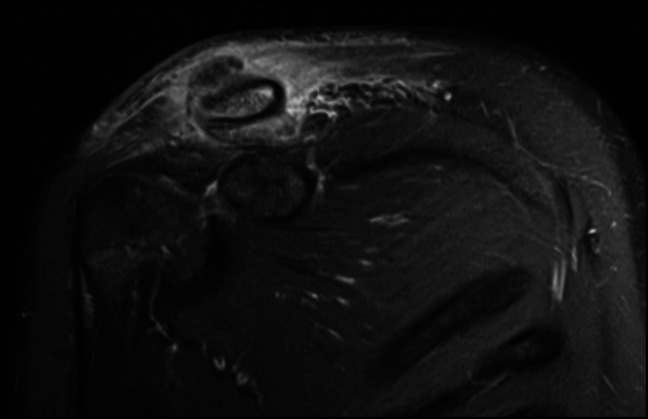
Coronal postcontrast T1-weighted MRI periosteal reaction with cortical erosion, periosteal elevation, and associated soft-tissue swelling.
